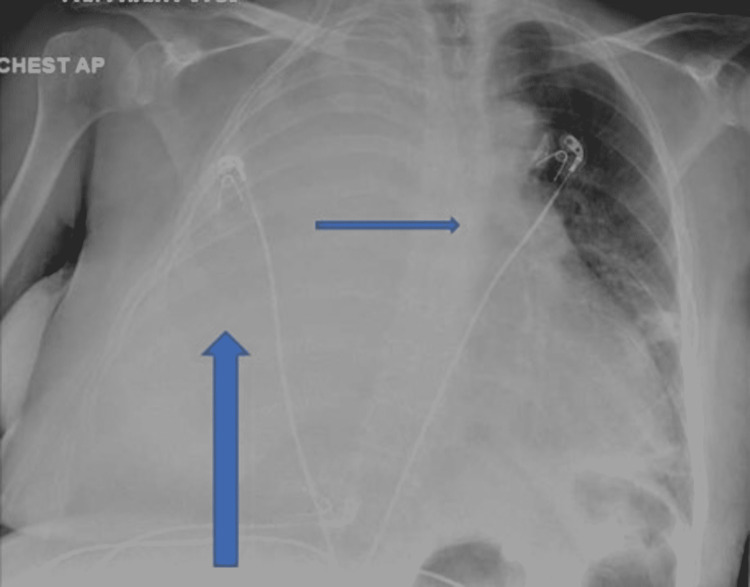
Portable chest X-ray anteroposterior view showing almost complete opacification of the right hemithorax with a mediastinal shift to the left (blue arrows).
